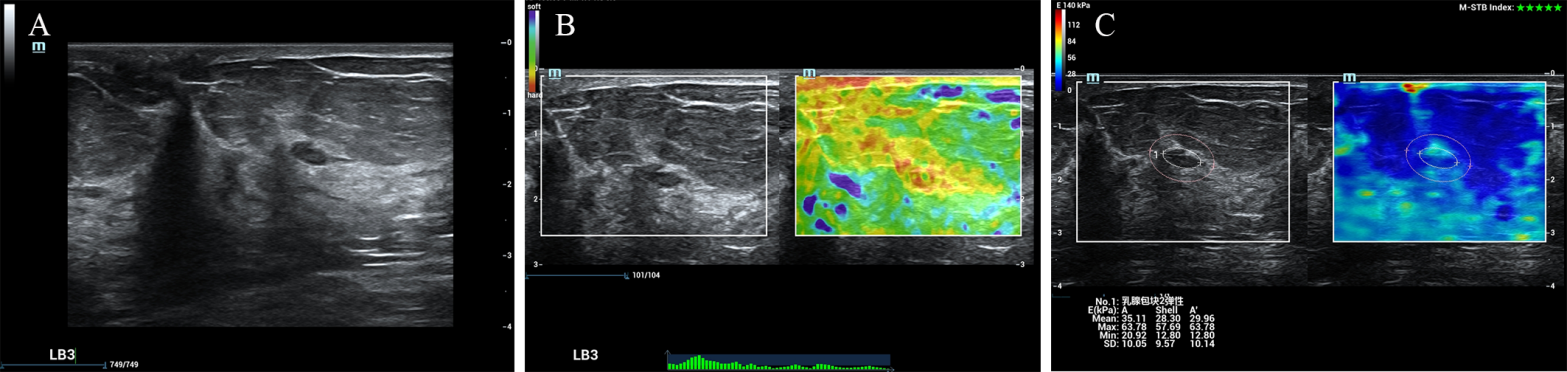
The breast cancer was downgraded by the Emax-A’-S2.5 below 80kpa. A 65-year-old female patient had a lesion on her left breast. Pathologically confirmed intraductal solid papillary carcinoma. The size of the lesion was 6.6mm and ultrasound images of the breast lesion were evaluated as BI-RADS 4a (A), with an elasticity score of 5 (B), Emax-A of 63.8kpa and Emax-A’-S2.5 of 63.8kpa (C).
